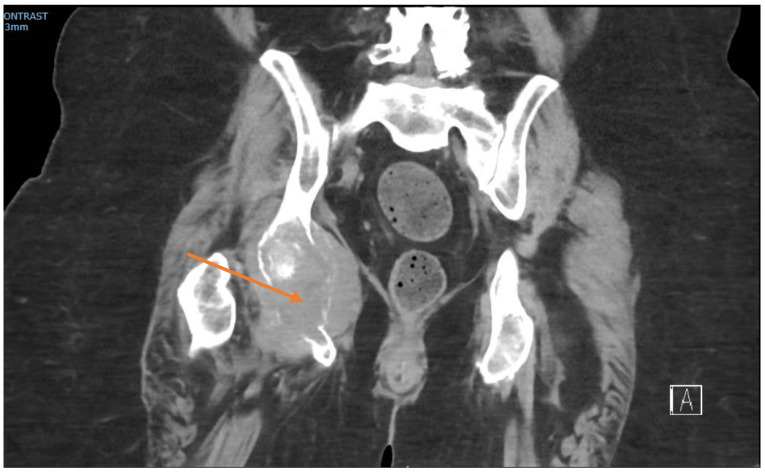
Coronal CT pelvis showing lytic destruction of the right ischium (arrow), with soft-tissue mass extension.
